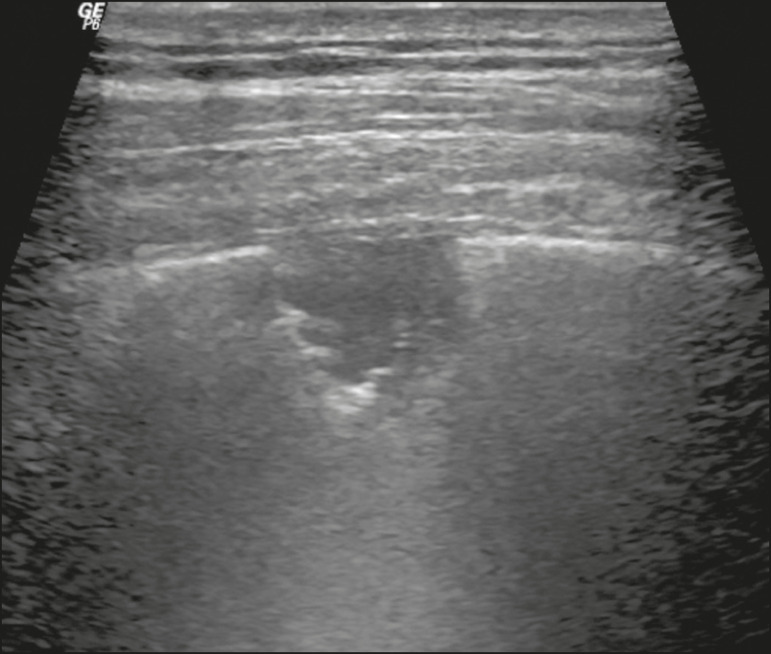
Chest ultrasound in a patient with COVID-19. The presence of pleural thickening, a hypoechogenic image with irregular contours, compatible with subpleural consolidation, less than 2.0 cm, and discrete C-lines are identified with the linear transducer in the intercostal space.
